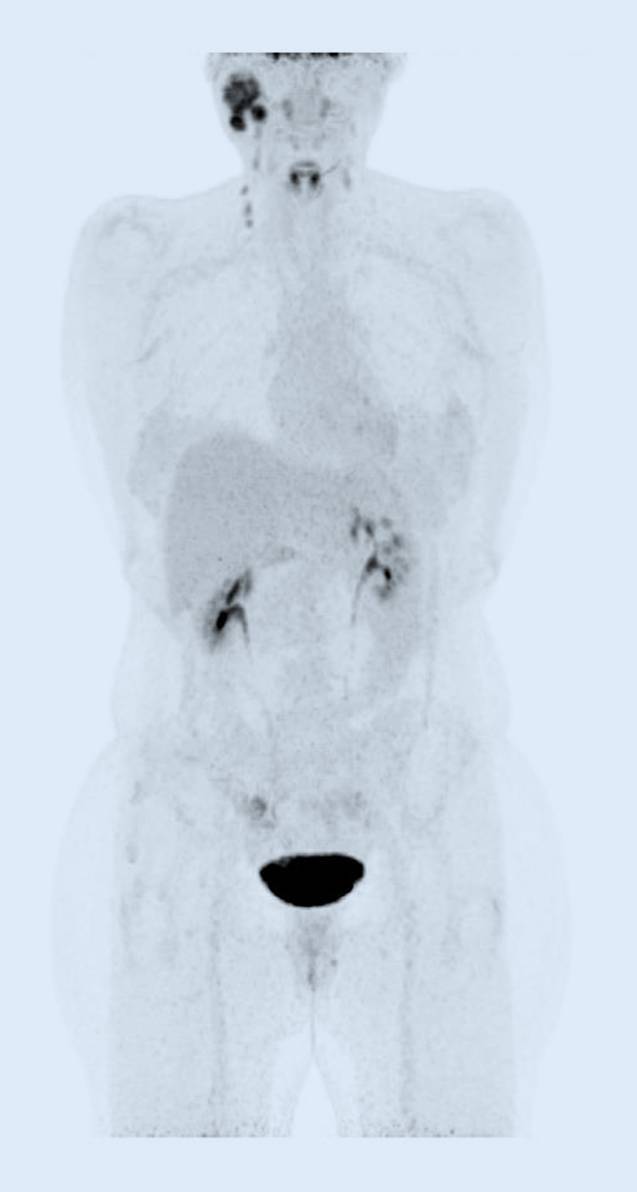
Ganzkörper-18-FDG-PET mit Primärtumor und Halslymphknotenmetastasen, ohne Nachweis von Fernmetastasen vom Juni 2023
